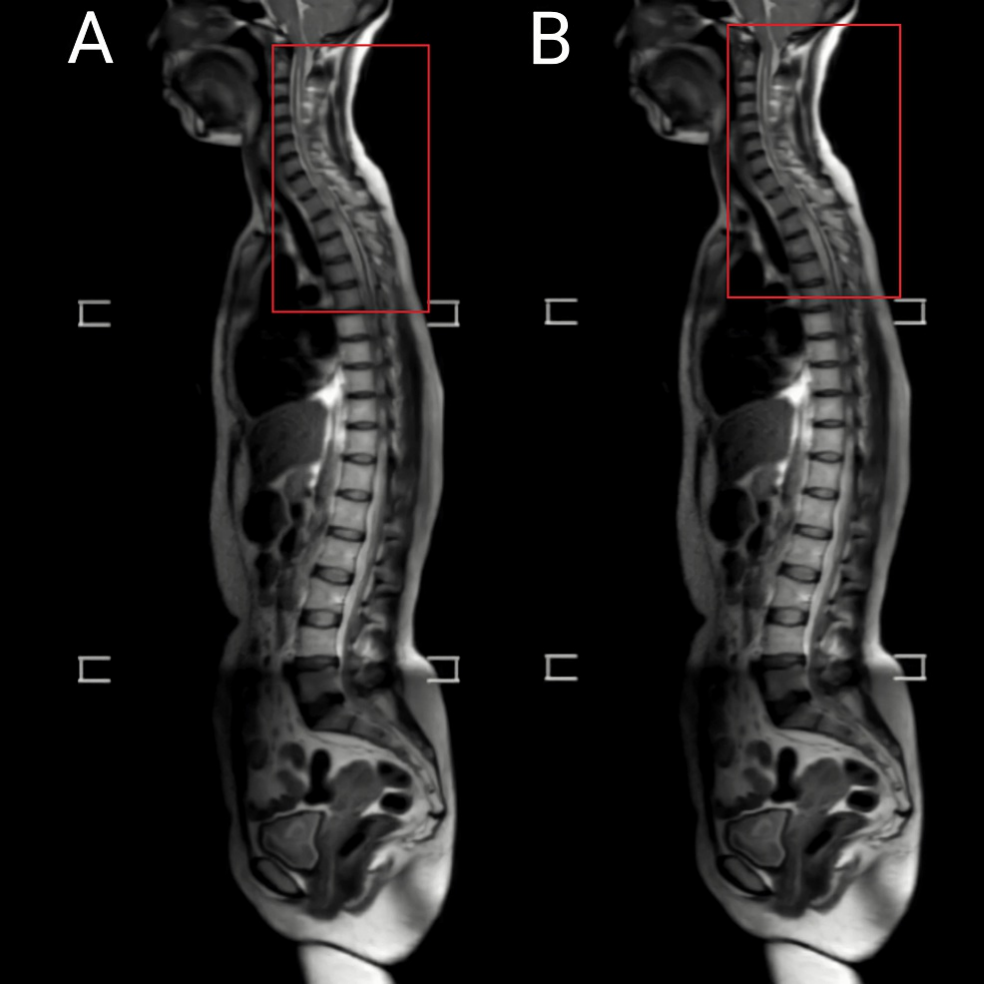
MRI shows intramedullary T2WI/STIR hyperintensity noted in spinal cord from C2- D10 vertebral levels suggestive of syrinx
